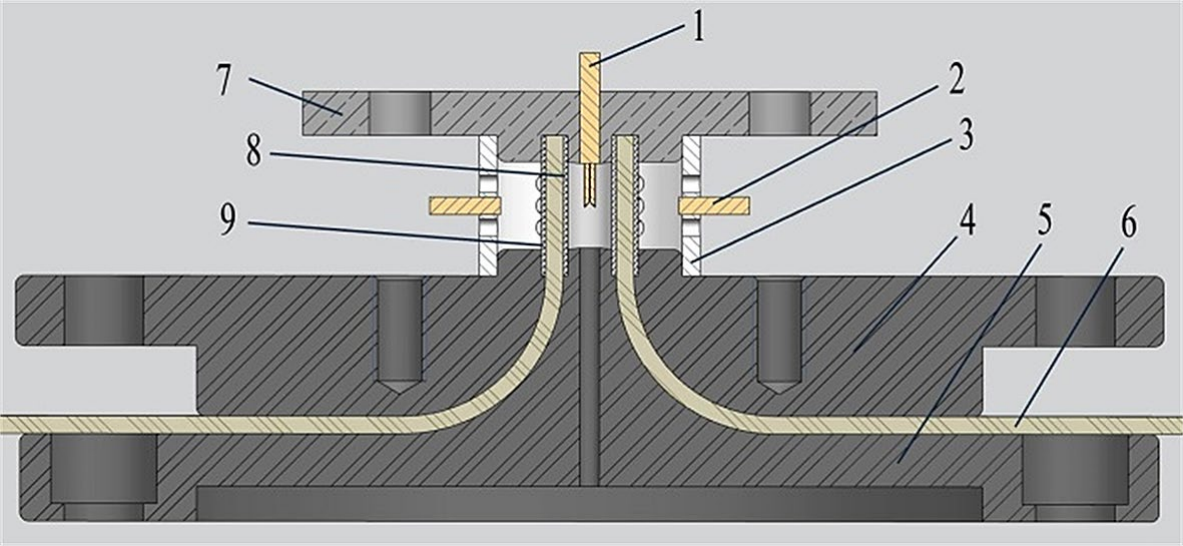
Installation layout of the load configuration in CQ3-Shot137. (1. internal photonic Doppler velocimetry probes 2. external photonic Doppler velocimetry probe 3. probe support 4. anode flange 5. cathode flange 6. high-density polyethylene insulation 7. short-circuit cap 8. inner liner 9. outer liner).
